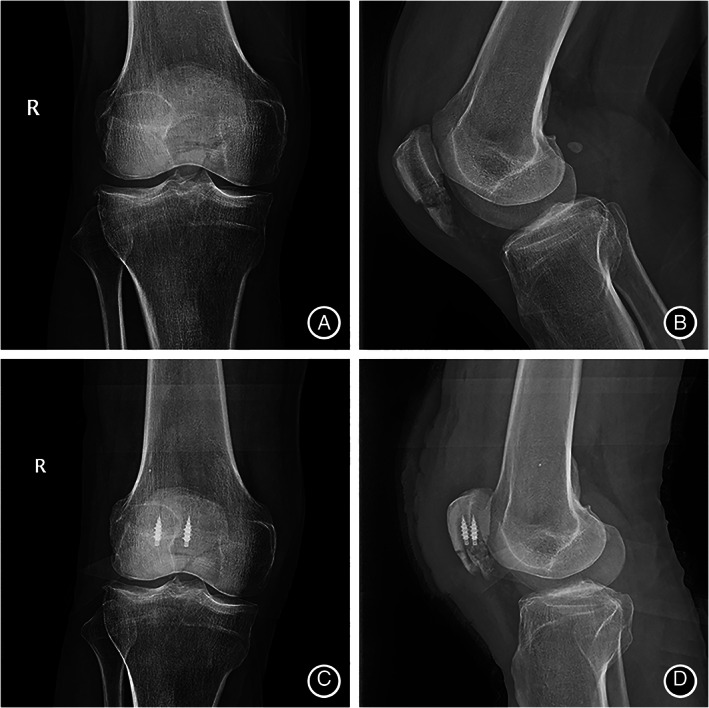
Anterioposterior and lateral knee radiographs of a 52‐year‐old male patient (A,B) Patients with distal pole patellar fractures; (C,D) after treatment with anchor and Krackow‐“8” suture fixation.
